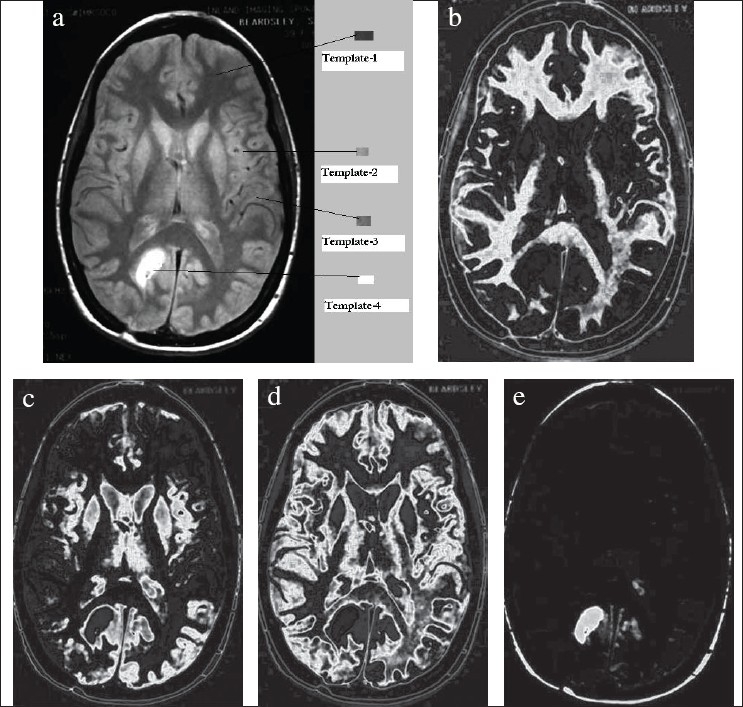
(a) Original brain CT image marked with templates, (b) Segment-1 corresponding to template-1, (c) Segment-2 corresponding to template-2, (d) Segment-3 corresponding to template-3, (e) Segment-4 corresponding to template-4
